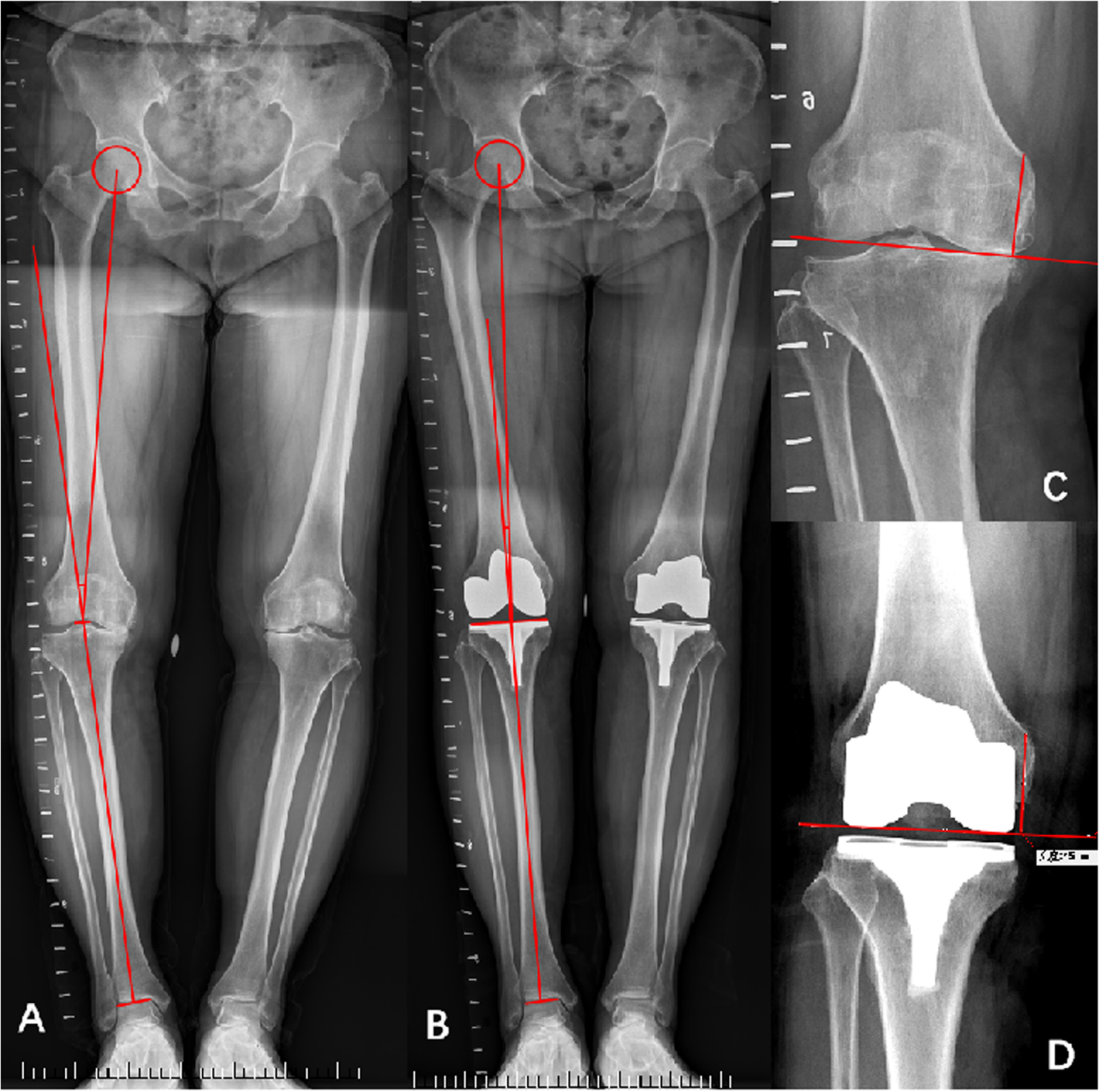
The lower extremity long-standing radiograph and positive X-ray with preoperative and postoperative alignment (red line). HKA is defined as the angle between a line from the femoral head center to the tibial spine center and a line from the tibial spine center to the talus joint surface centre (a, b). The method of measuring the change of joint line before and after operation with the insertion point of the adductor femur as the reference point (c, d)
